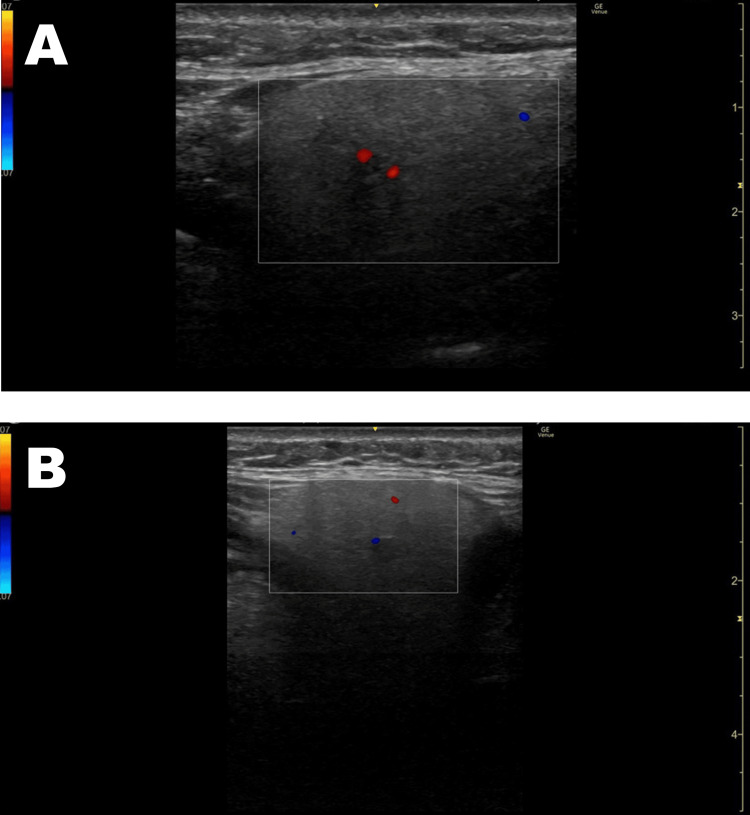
Neck color Doppler ultrasound findings (11 months after CIS recurrence). (A) Right submandibular gland. (B) Left submandibular gland Follow-up neck color Doppler ultrasound showed no salivary gland enlargement, tubular structures, or increased blood flow.
